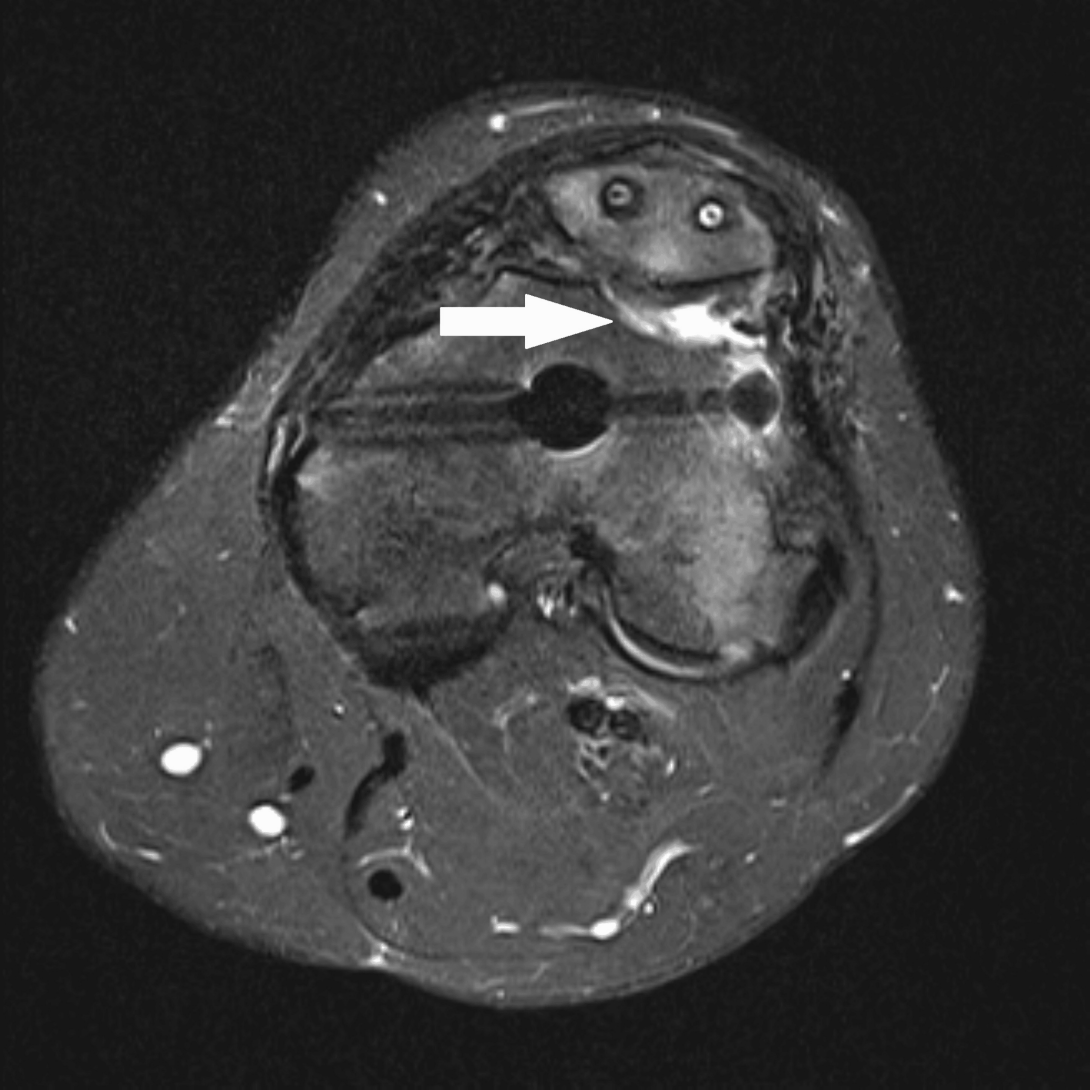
Pre-operative axial cut of short tau inversion recovery (STIR) MRI of the left distal femur demonstrating mild lateral patellar subluxation with thickening, intermediate signal of the patellar retinaculum, and lateral patellofemoral chondromalacia, as highlighted by the arrow.
